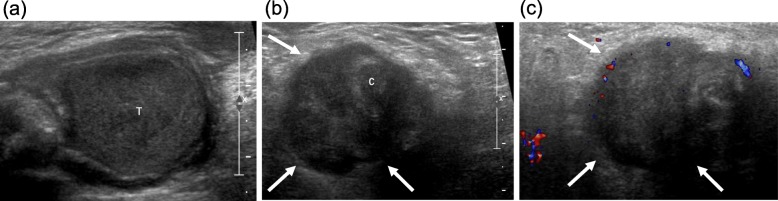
Scrotal ultrasonography images. a Right testis (T) with normal contour but decreased echogenicity. b A hypoechoic mass (arrows) above the right testis, surrounding the spermatic cord (C). c No increased blood flow in the right testis and the hypoechoic mass (arrows) on color Doppler ultrasonography (CDUS)
